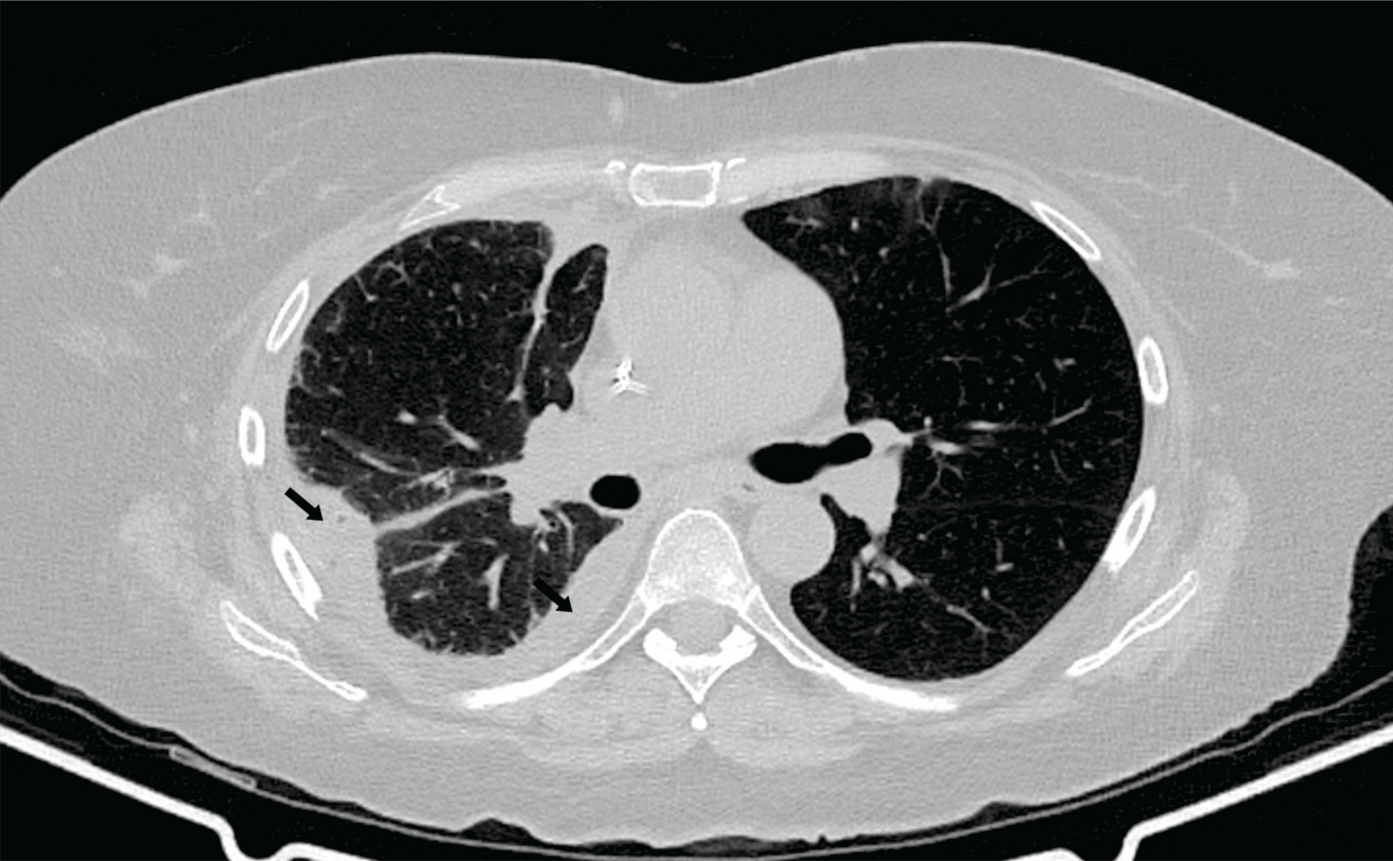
Chest CT one month after discharge Chest CT was performed one month after discharge. A decrease in effusion size (black arrows) is seen with no new consolidations.
